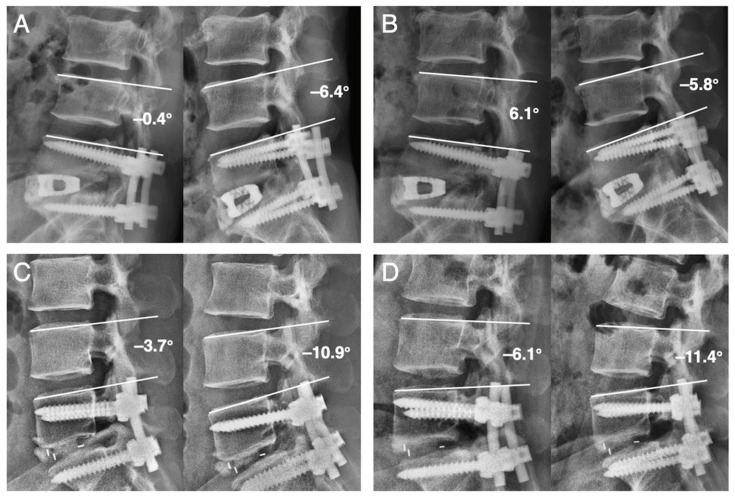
Dynamic lateral radiographs exhibit examples of development/non-development of instability (angular motion > 10°) at L4-L5 in patients who received ALIF or TLIF treatment. Images were obtained from a 48-year-old man who received L5–S1 ALIF (A,B) and a 41-year-old woman who received L5–S1 TLIF (C,D). (A) Postoperative radiographs exhibiting normal angular motion at L4–L5 (6°). (B) 12-month follow-up radiographs showing 11.9° angular motion at L4–L5 with definitive evidence of instability. (C) Postoperative radiographs showing normal angular motion at L4–L5 (7.2°). (D) 12-month follow-up radiographs exhibiting 5.3° angular motion at L4–L5 without definitive evidence of instability.
